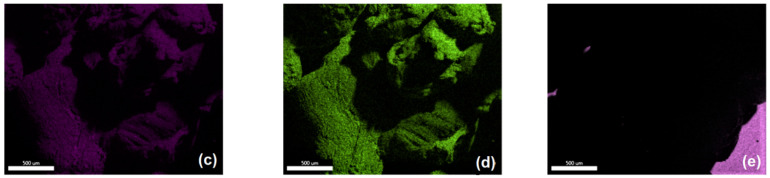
SEM-EDS images of PLA: (a)—SEM image of PLA and X-ray maps of (b)—carbon, oxygen, silicon; (c) carbon; (d)—oxygen; (e)—silicon.
